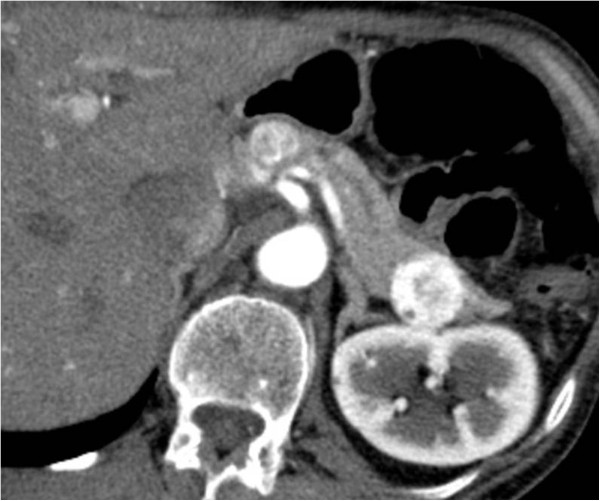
Multiple lesions. An arterial phase acquisition on CT shows two synchronous metastases as inhomogeneously enhancing nodular masses in the pancreatic body and tail.
